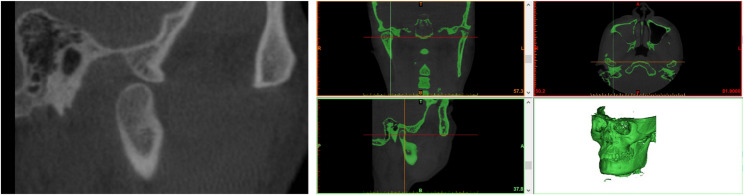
3D reconstruction of CBCT using mimics software
